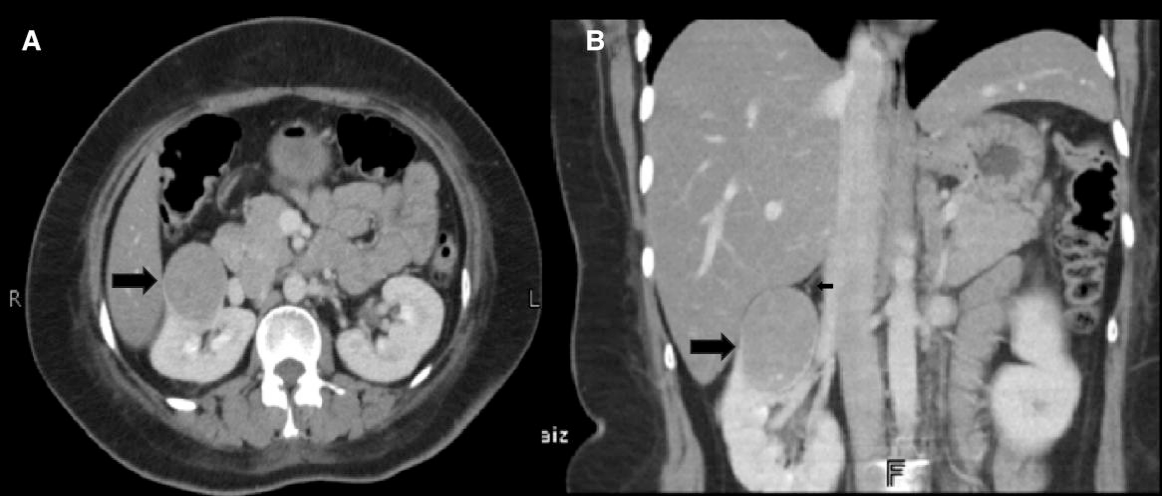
A 4-cm, round, well-circumscribed mass located in the upper pole of the right kidney, distinct from the adrenal gland on A, axial and B, coronal computed tomography of the abdomen.
